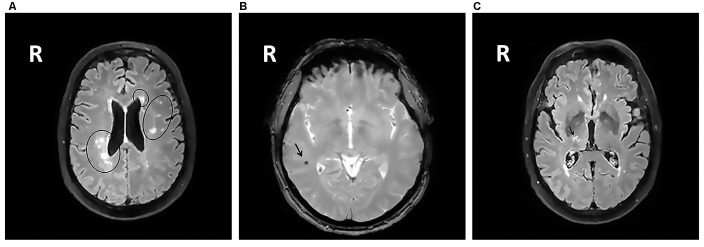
(A) Fluid-attenuated inversion recovery (FLAIR) brain magnetic resonance imaging (MRI) scan showing periventricular WMHs and deep WMHs (DWMHs) in both hemispheres. WMH, white matter hyperintensities; R, right. (B) T2*-weighted brain MRI scan showing a microbleed located within the right white matter. R, right. (C) FLAIR brain MRI scan showing a lacune located in the deep gray matter. R, right.
